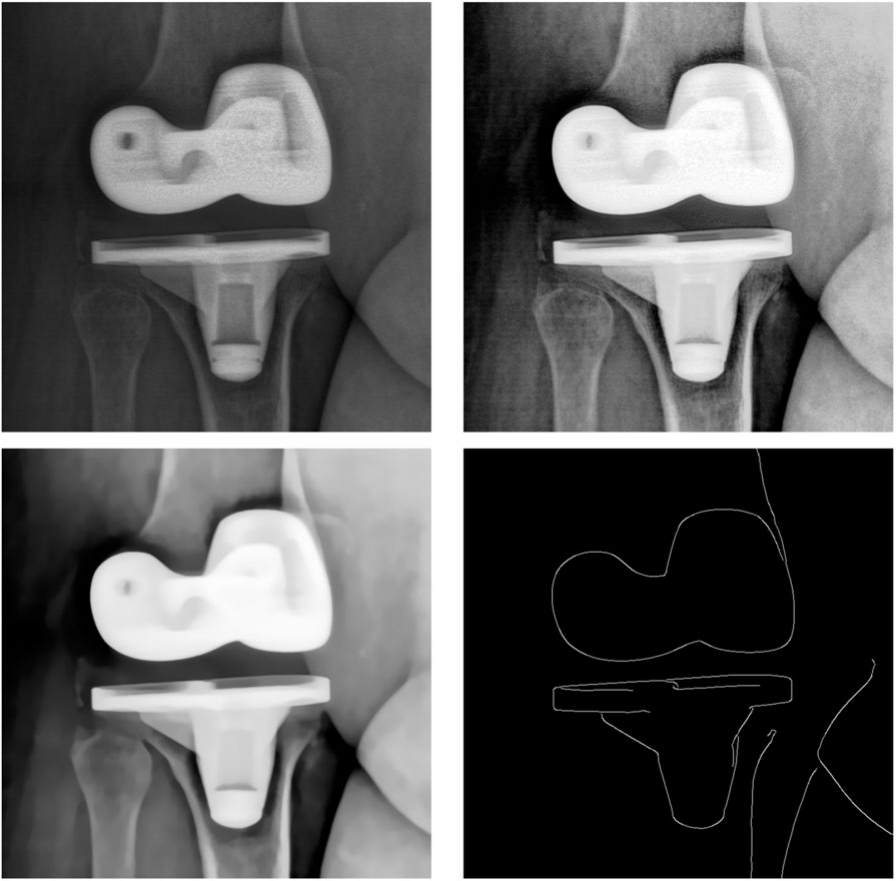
Example of contour-based pre-processing step. From left to right, first row : input X-ray image, histogram equalization image enhancement, and second row : denoised image, pre-processed image
